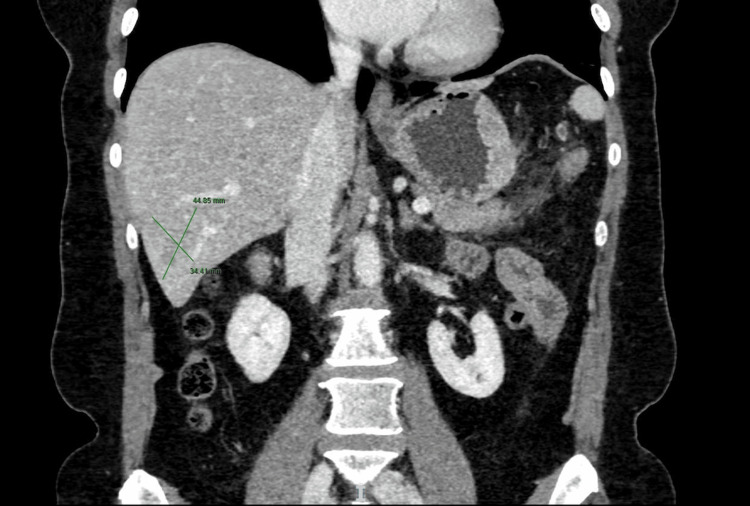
Preoperative abdominal CT scan (venous phase, coronal plane): a liver metastasis with undefined margins involves segments VI and VII CT: Computed Tomography
